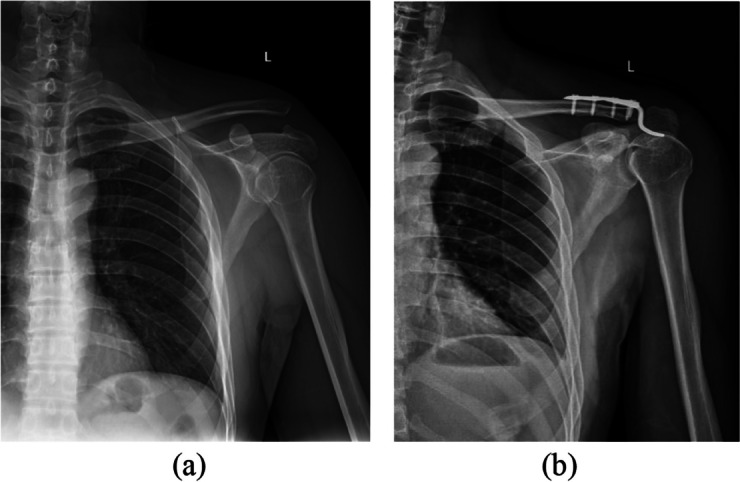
Basic information: male, left ACJ dislocation,(Rockwood type III), treatment with clavicular hook plate technique. a the preoperative positive X-ray film showed that the distal clavicle was raised and the space between ACJ and CCJ was widened; b the postoperative positive X-ray film showed that the reduction of the left ACJ was good
